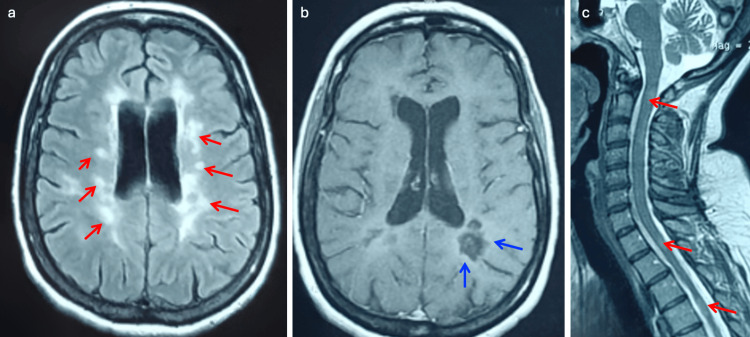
Brain (axial T2-FLAIR (a) and post-contrast T1 (b)) and cervical spinal cord (sagittal T1 (c)) MRI revealing multiple demyelinating lesions, including an enhancing lesion in the right centrum semiovale FLAIR: fluid-attenuated inversion recovery
